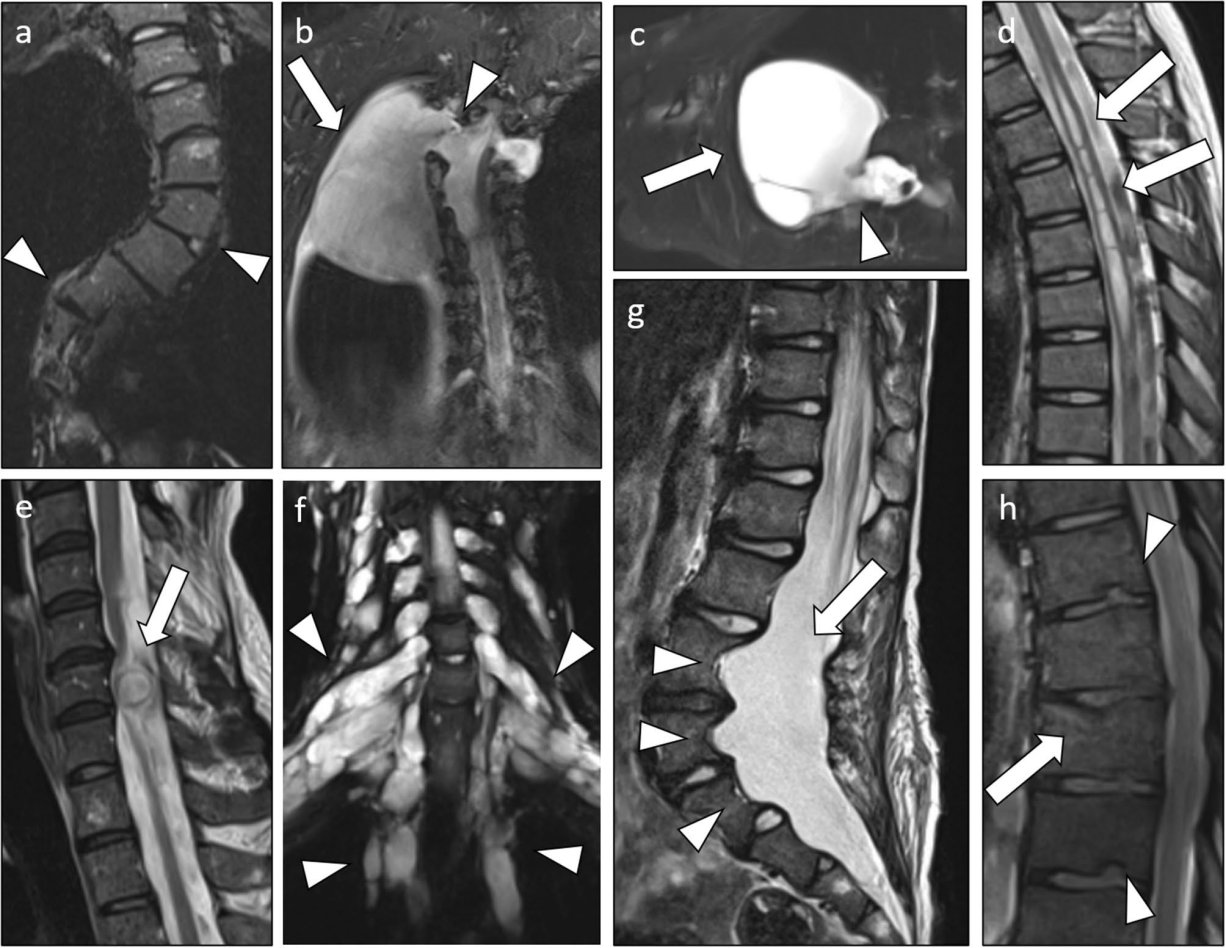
T2-weighted MRIs of investigated spinal abnormalities and tumors in patients with Neurofibromatosis type 1. Displayed are: A coronal image of a biconvex scoliosis of the thoracal spine (a). Coronal (b) and axial (c) images of a large meningocele (arrow) protruding through the enlarged neuroforamen of Th3 (arrowhead) into the thorax. A sagittal image of a septate thoracal syringomyelia (arrows) (d), a sagittal image of an intraspinal tumor at level C6 with compression of the spinal cord (e) and a coronal image of a patient with large paraspinal plexiform neurofibromas (arrowheads) growing along the whole spine (f). A sagittal image of a large dural ectasia (arrow) with scalloping of the lumbar vertebrae (arrowheads) (g) and a sagittal image of a vertebral fracture (arrow) and herniations of intervertebral discs into adjacent vertebrae (arrowheads) (h).
